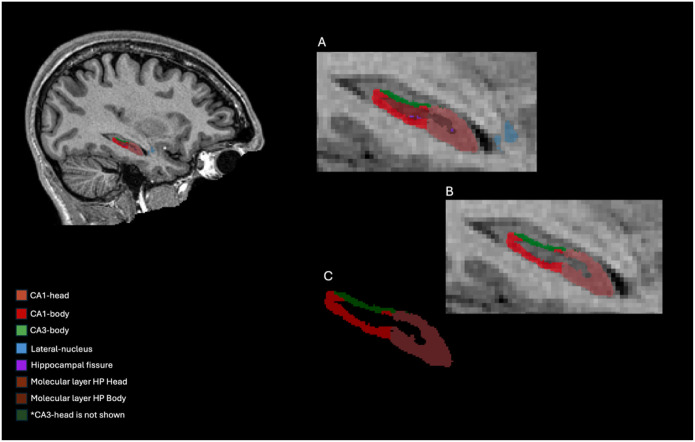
FreeSurfer Hippocampal segmentation. A. Sagittal view segmentation of the whole hippocampus with subject’s MRI for reference B. Sagittal view segmentation of relevant regions (CA1 head/body and CA3 head/body) with subject’s MRI for reference C. Sagittal view segmentation of relevant regions (CA1 head/body and CA3 head/body) without the reference image
